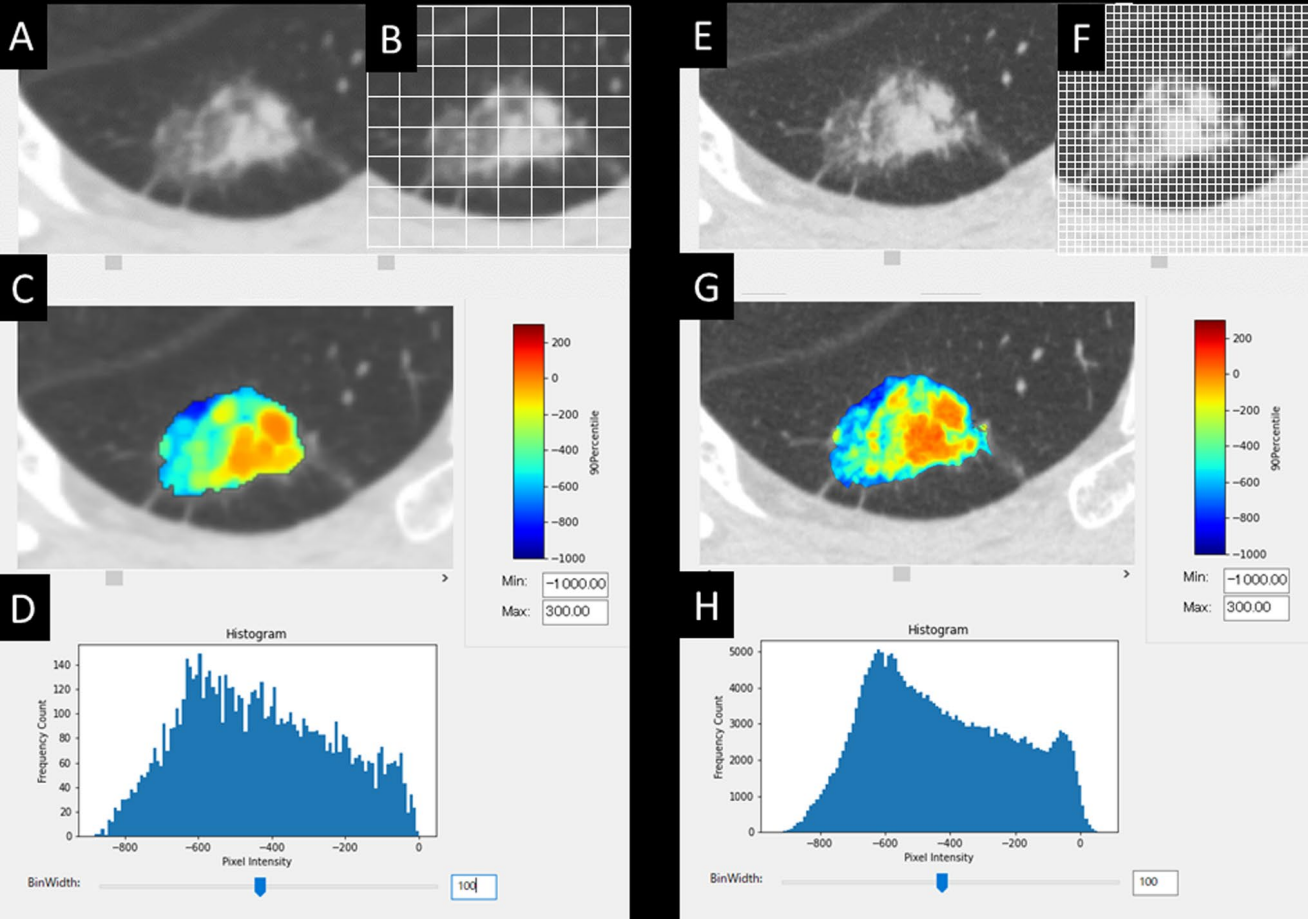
Comparisons of CT histograms and color mapping of radiomics features between NSR- and HSR-models. 71-year-old woman with a part-solid nodule measuring 1.5 cm in total diameter and 0.3 cm in solid component diameter (pathological T descriptor: pT1mi). A Normal-spatial-resolution (NSR) image. B 512 matrix. C Color mapping of radiomics feature from NSR image. D CT histograms obtained from the volumetry using NSR data. E High-spatial-resolution (HSR) image. F 2048 matrix. G Color mapping of radiomics feature from HSR image. H CT histograms obtained from the volumetry using HSR data
